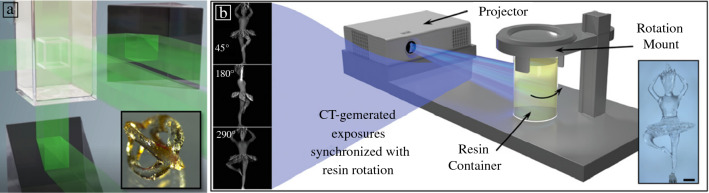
(a) Holographic volumetric additive manufacturing (VAM)—uses overlap of perpendicular projections. (b) Tomographic VAM—uses projection of computed tomography (CT)-generated exposures into a rotating resin vat. Images reused with permission.^68,69^
